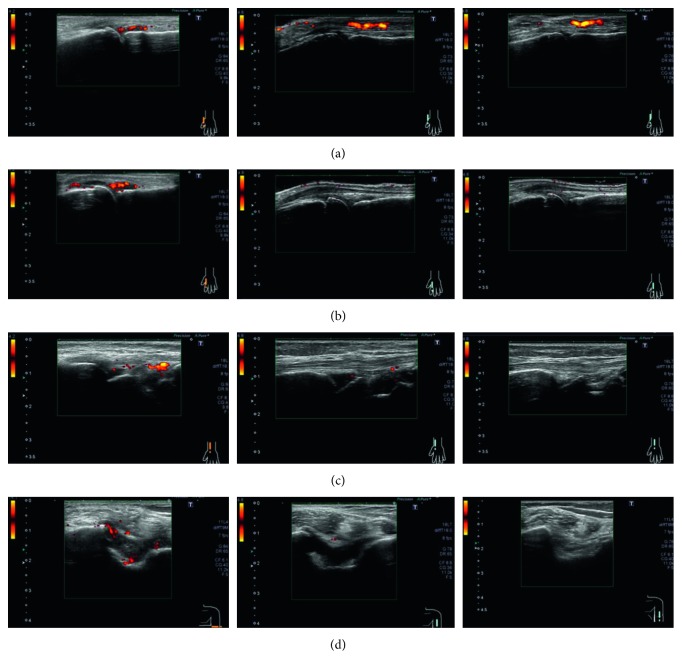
Joint ultrasonography findings. Left, before baricitinib administration; center, 24 weeks after the start of baricitinib administration; right, 52 weeks after the start of baricitinib administration. The abnormal findings in the following joints disappeared for up to 52 weeks in both PD and GS examinations: (a) left first MCP joint, (b) left second MCP joint, (c) left radial joint, and (d) left elbow joint (extensor surface/cross-sectional view). GS: grayscale; PD: power Doppler; MCP: metacarpophalangeal.
